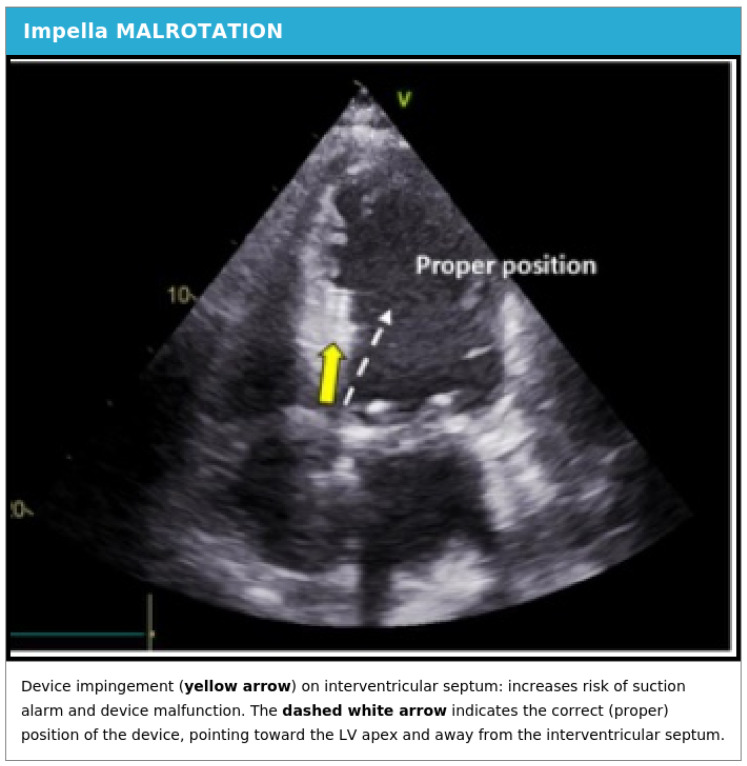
Echocardiographic evaluation of Impella malrotation.
